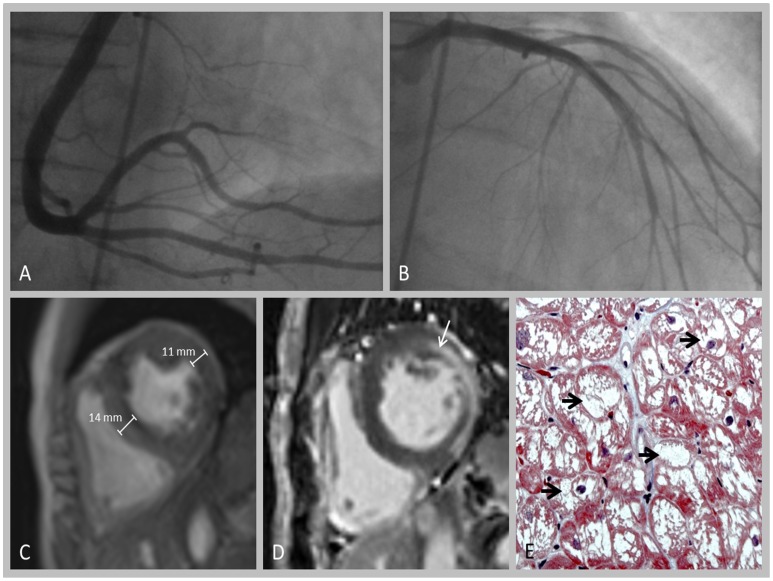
Cardiac work up in patient 2. A+B: Coronary angiography (A: right coronary artery; B: left coronary artery) demonstrating no relevant pathology. C+D: Cardiac MRI showing increase in myocardial wall thickness (C) and pathological late gadolinium enhancement (D, arrow). E: Myocardial biopsy revealing strong accumulation of Gb_3,_ as indicated by numerous vacuoles within the cardiomyocytes (arrow).
